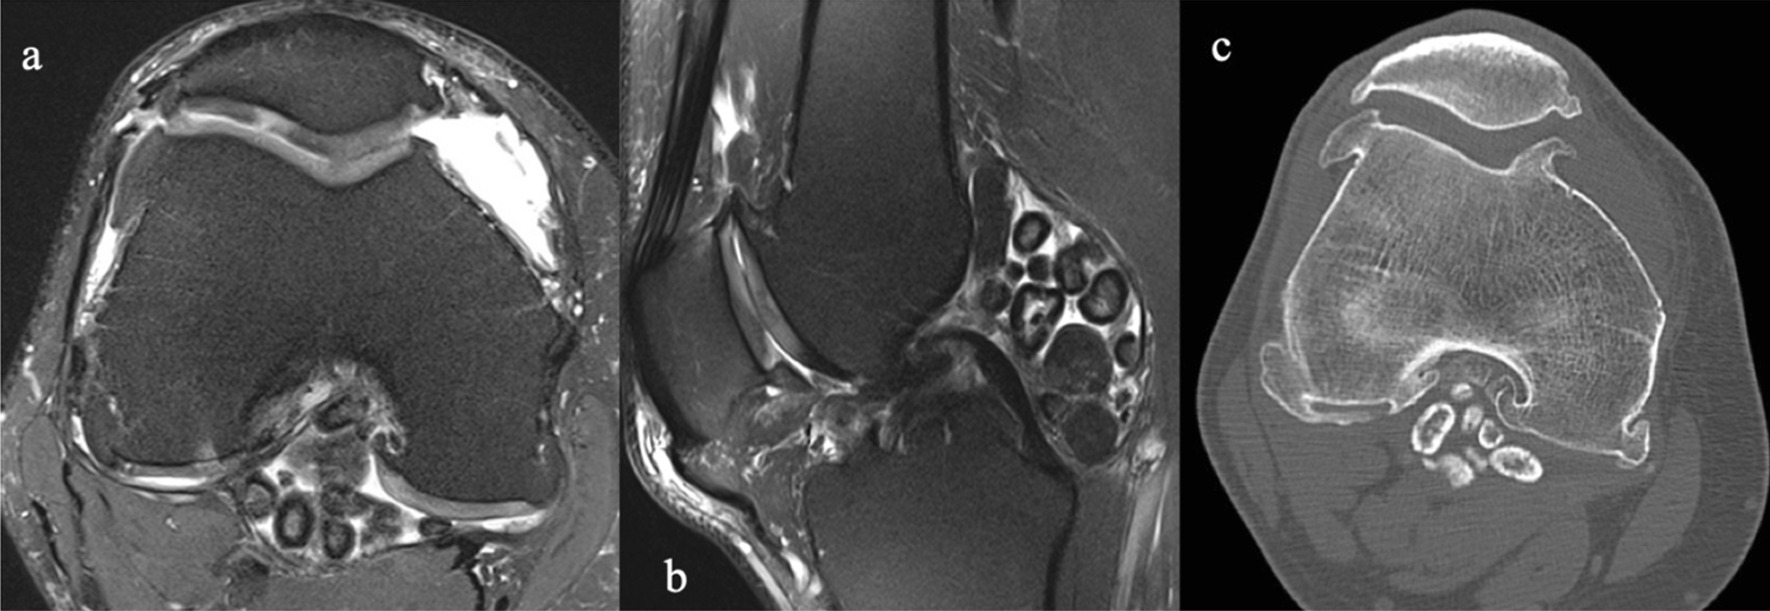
a MRI axial view and b MRI sagittal view. Chondropathy, minimal synovial hypertrophy areas, multiple osteophytes in the intercondylar notch. c Computed tomography axial view: posterior loose bodies and multiple intercondylar notch osteophytes
